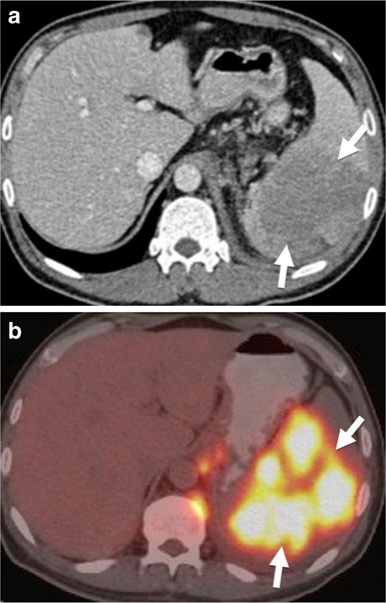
Splenic lymphoma. (a) Axial contrast-enhanced CT of a 30-year-old man reveals a large hypodense mass in spleen (*arrows*) (b) Increased FDG uptake is also noted (*arrows*)
